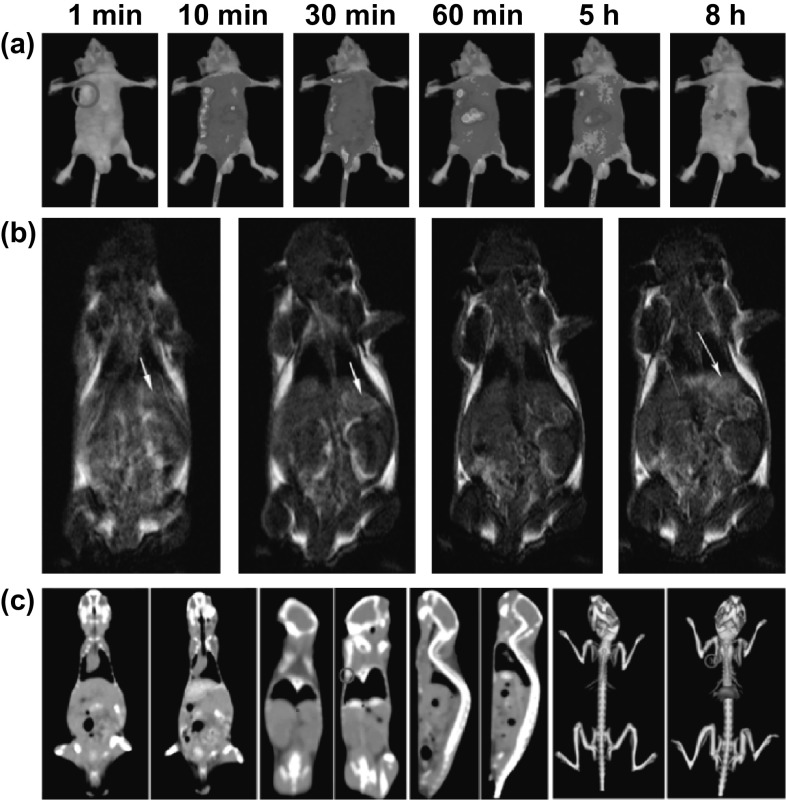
In vivo trimodal bioimaging. a PL images at different time points. b T_1_-weighted MRI coronal images. c CT coronal view images. Reproduced with permission from Ref. [106]. Copyright 2016 American Chemical Society
